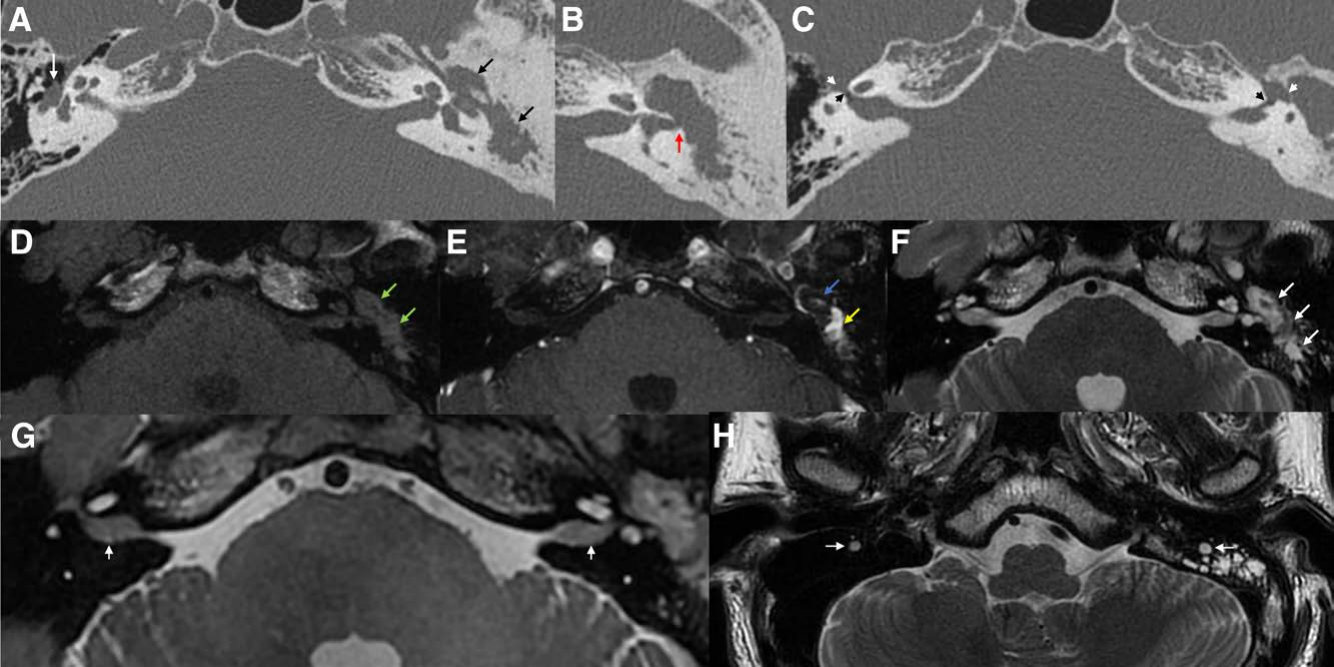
(A–C) Axial view of temporal bone computed tomography. (A) Total opacification of the left middle ear and mastoid cavity (black arrows) with sclerotic changes of the mastoid bone. Prominent soft tissue is noted at the inferior aspect of the mid-tympanic course of the right facial nerve (white arrow). (B) Focal dehiscence of the left lateral semicircular canal was suspected (red arrow). (C) Widening of the bilateral facial nerve canal at the labyrinthine (black arrowheads) and tympanic segment (white arrowheads). (D–F) Axial view of IAC MRI. (D) Precontrast T1-weighted image shows iso-signal intensity lesion in the left middle ear and mastoid cavity (green arrows). (E) Postcontrast T1-weighted image shows enhancing (yellow arrow) and non-enhancing (blue arrow) soft tissue lesion. (F) T2-weighted image shows high signal intensity lesion in the left mastoid and middle ear cavity (white arrows). Axial view of 3-dimensional proton density-weighted image demonstrates enlargement of the canalicular segment (white arrows, G) and mastoid segment (white arrows, H) of bilateral facial nerves.
